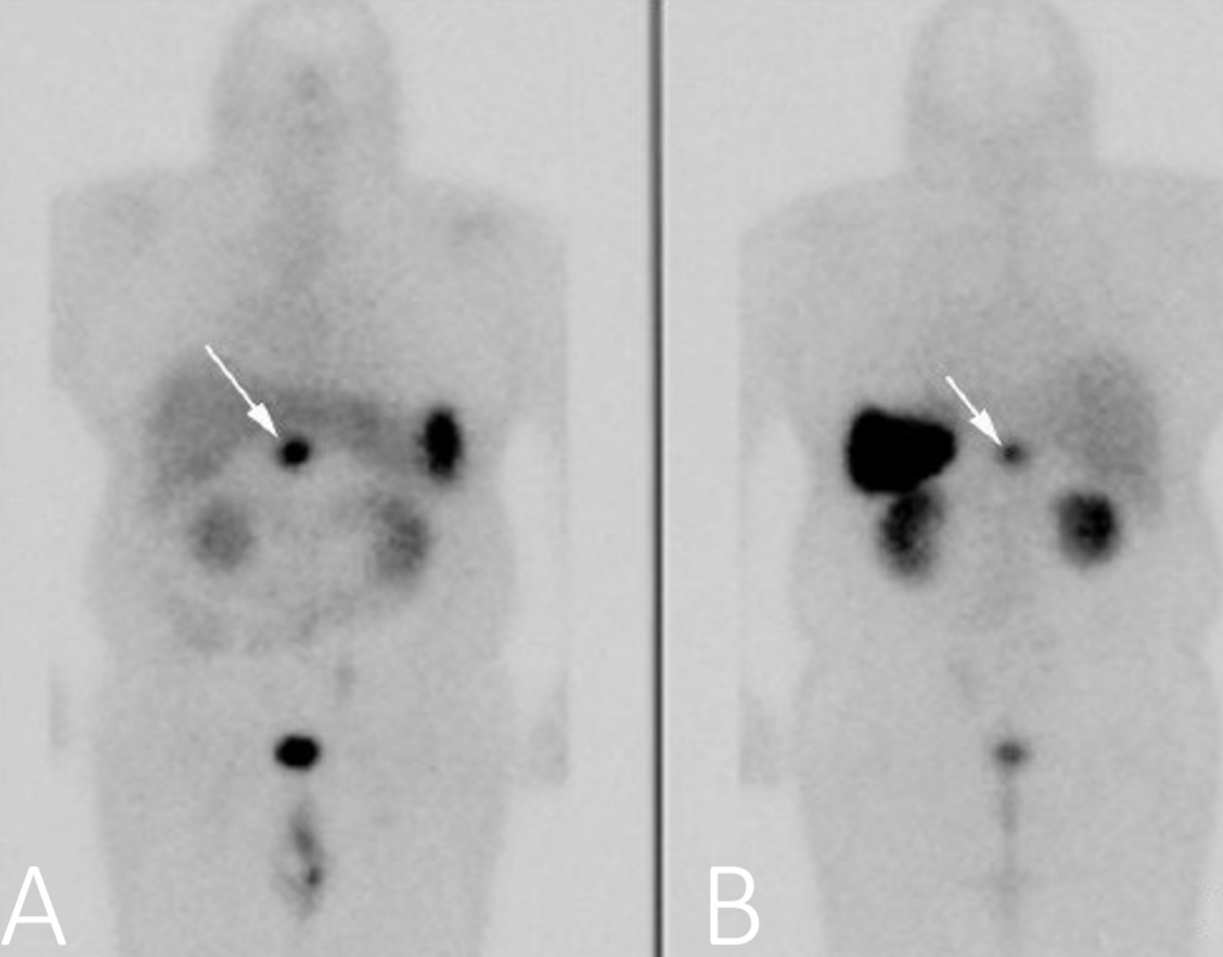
Somatostatin receptor scintigraphy: anteroposterior (A) and posteroanterior (B) view four hours after contrast administration demonstrating intense focal uptake in the area of the third portion of the duodeunum. Also note the physiologic uptake of contrast into the spleen, kidneys, and bladder.
